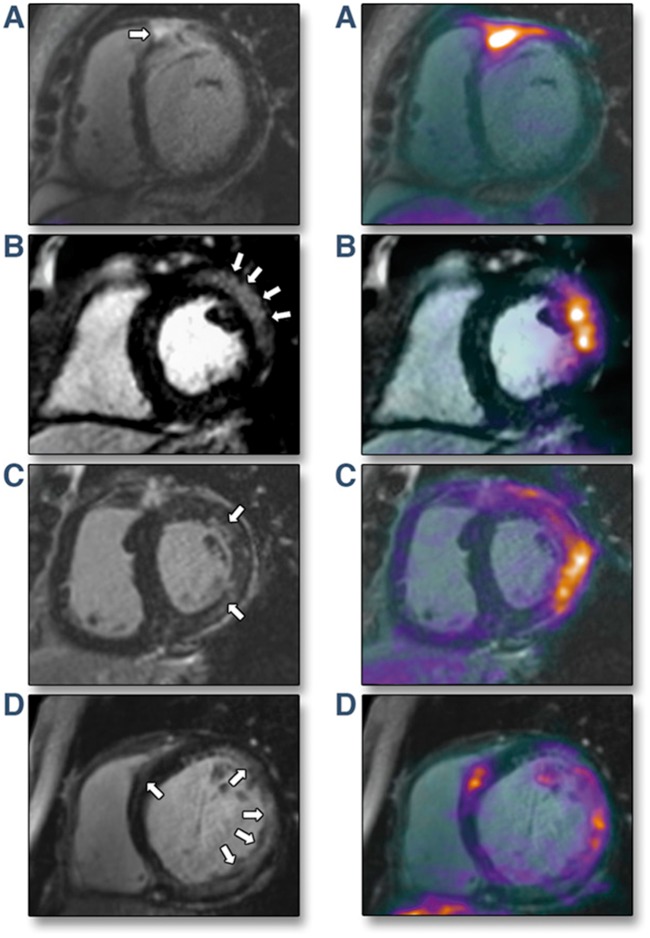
Imaging active cardiac sarcoidosis. *Left panel:* Late gadolinium enhancement (LGE) MRI. *Right panel*: Hybrid LGE and ^18^F-fluorodeoxyglucose (^18^F-FDG). (*A*) Subepicardial LGE in basal anteroseptum extending into right ventricular free wall and increased ^18^F-FDG uptake at same region on fused PET-MR. (*B*) Subepicardial LGE in the basal anterolateral wall with increased ^18^F-FDG uptake co-localizing to identical region on PET-MR. (*C*) Patchy midwall LGE in anterolateral wall with matched increased ^18^F-FDG uptake on PET-MR. (*D*) Multifocal LGE in lateral wall with matched increased ^18^F-FDG uptake on PET-MR. Reproduced with permission from ref. 72.
